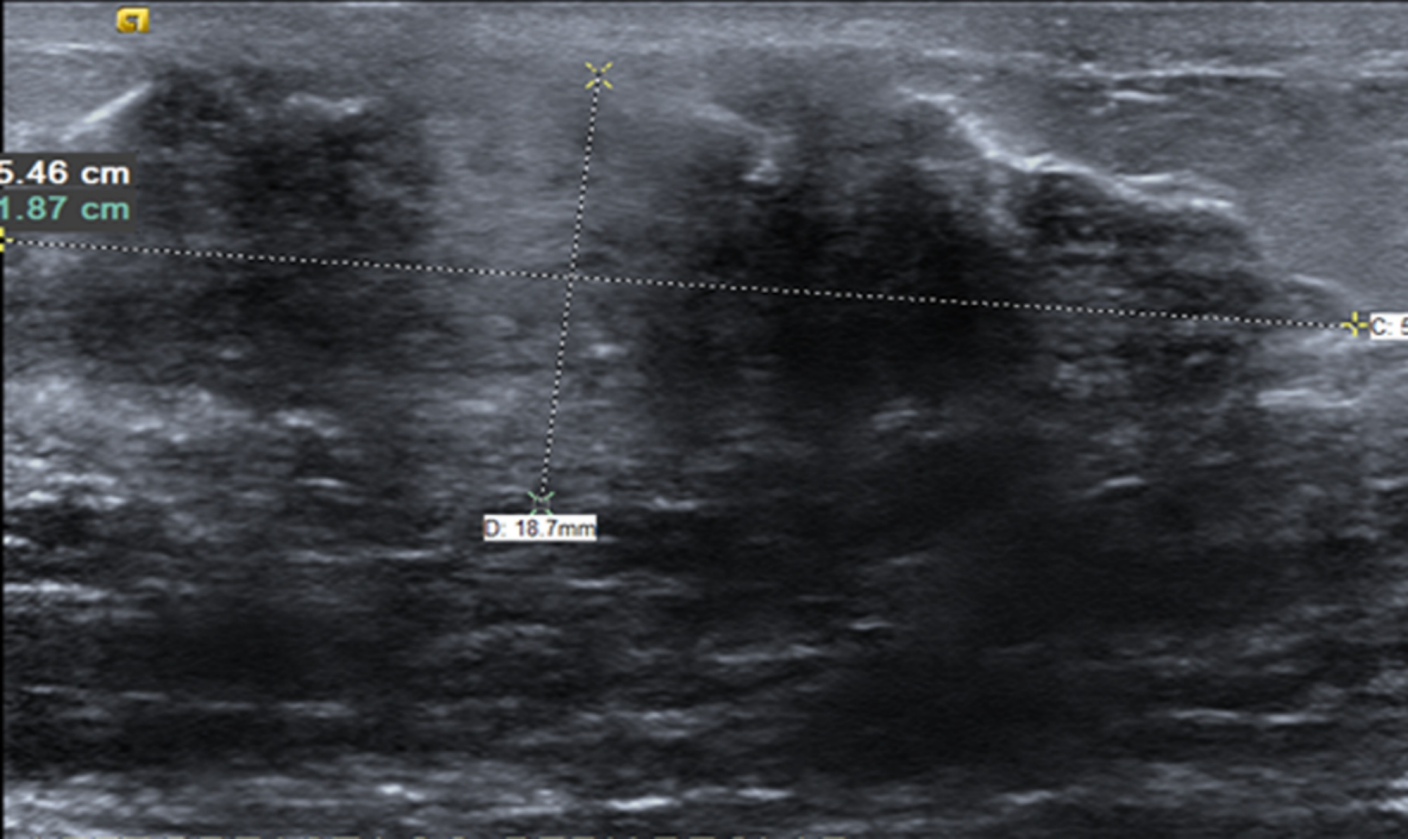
Ultrasound of the left breast Ultrasound of the left breast demonstrating a highly suspicious, ill-defined, deeply hypoechoic area of non-mass-like architectural distortion with acoustic shadowing, measuring 5.4×1.9 cm
